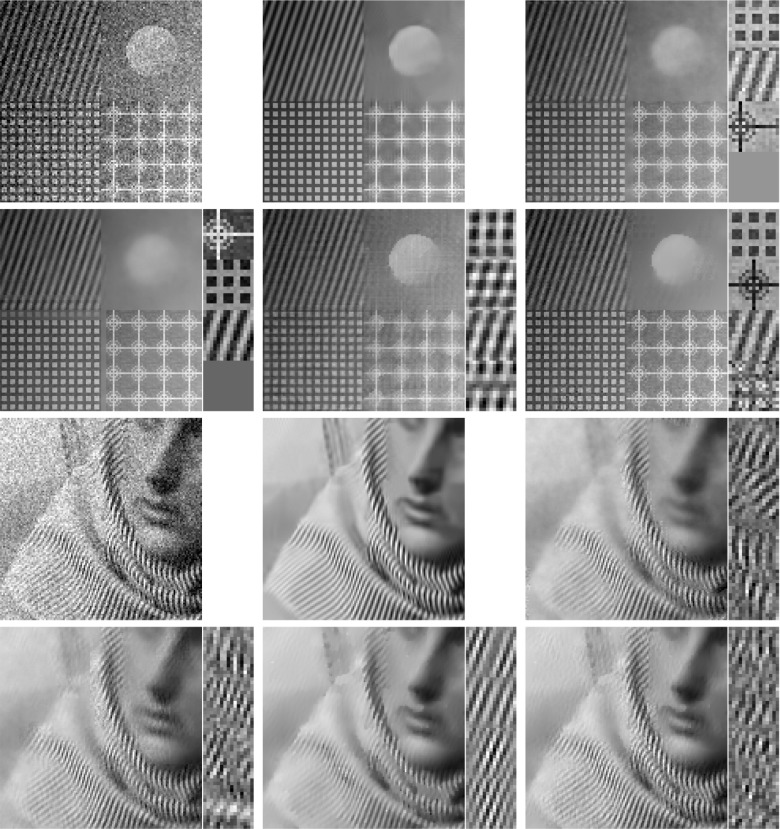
Comparison of different methods for denoising the *Patches* and *Barbara* images from Fig. 7. First row for each image, from left to right: Noisy data, BM3D, CDL. Second row for each image, from left to right: CL, CT-cvx and CT-scvx. The four most important learned atoms are shown right to the image, if applicable
